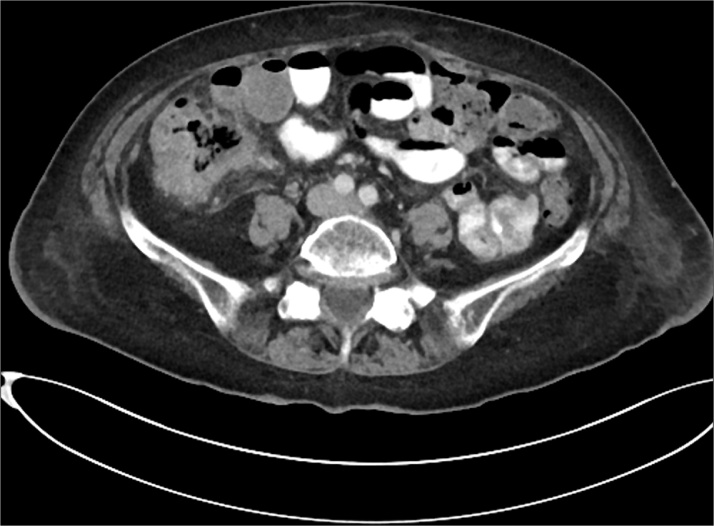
Admission CT scan demonstrating thick walled caecum with pericaecal inflammation.
